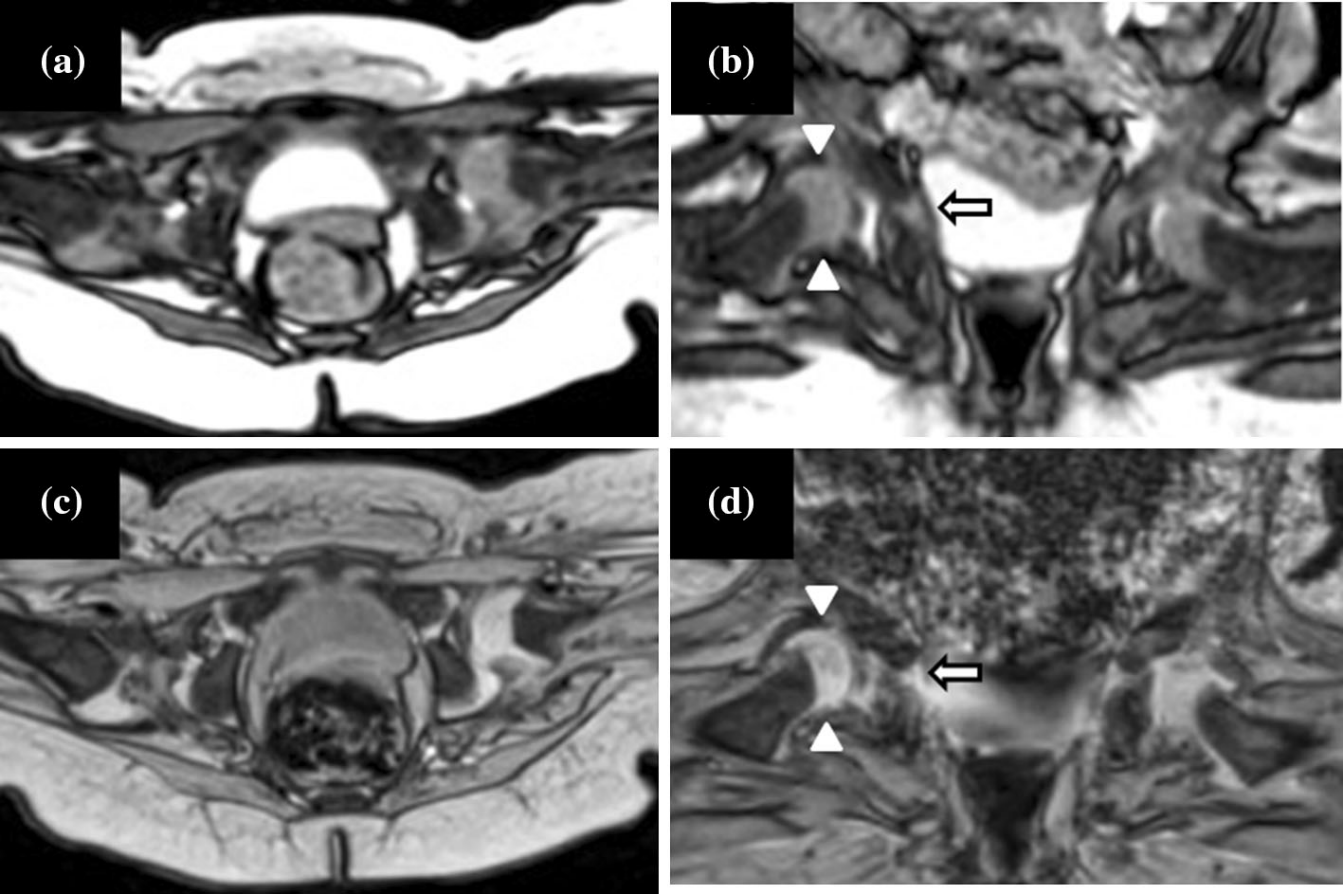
Transverse and coronal images by ultrafast (a, b) and conventional MRI (MEDIC) (c, d) of an incomplete reduction of the right hip and reduced left hip in an 8-month-old girl after the reduction procedure. No motion artefact was observed on ultrafast MRI. The results of the transverse and coronal images of the ultrafast MRI examination are similar to those of the conventional MRI examination. *White arrow* and *arrowheads* Right triradiate cartilage and incomplete reduction of the right hip, respectively. MEDIC Multiple-echo data image combination
